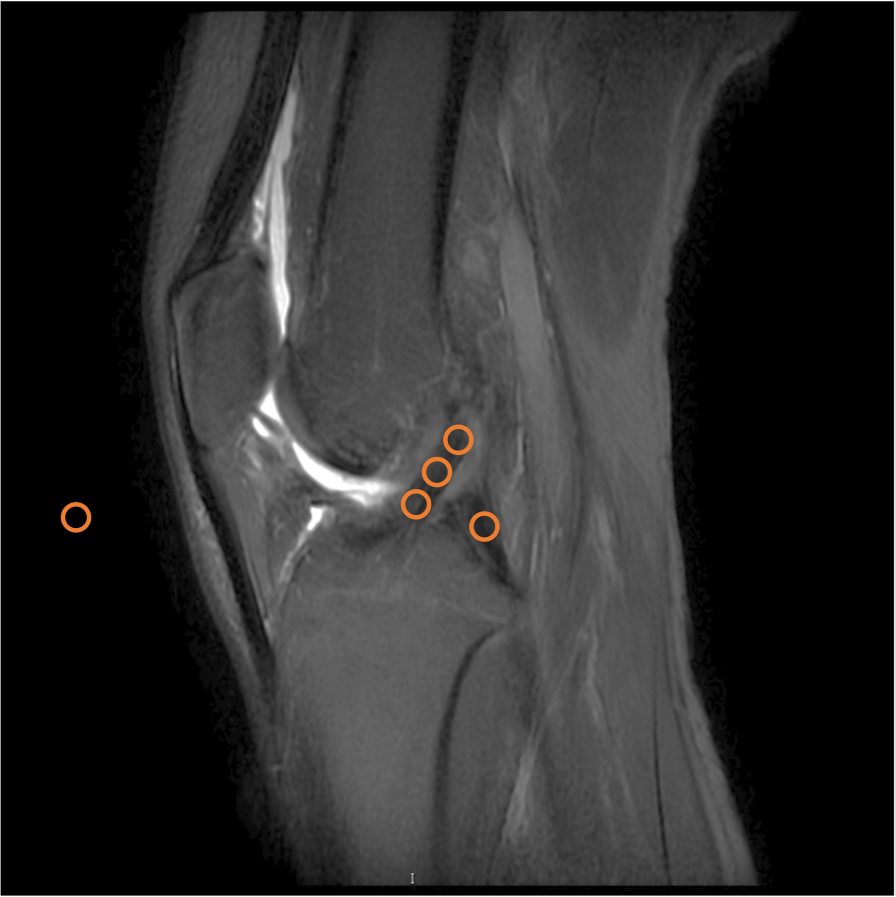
Measurement of SNQ. SNQ for ACL graft was calculated using the formula as followed: SNQ = (graft signal—PCL signal) / background signal. Signal intensity was measured with 15 ~ 20 cm^2^ circular ROIs on T2-weighted sagittal view. The graft signal was measured in its intra-articular portion at superior, middle, and inferior sites in the central slice of ACL. The average was calculated. The signal from the PCL was measured in its distal attachment. The background signal was measured 2 cm anterior to the patellar tendon via the same sagittal image slice. (*SNQ* signal/noise quotient, *ACL* anterior cruciate ligament, *PCL* posterior cruciate ligament, *ROI* regions of interest)
